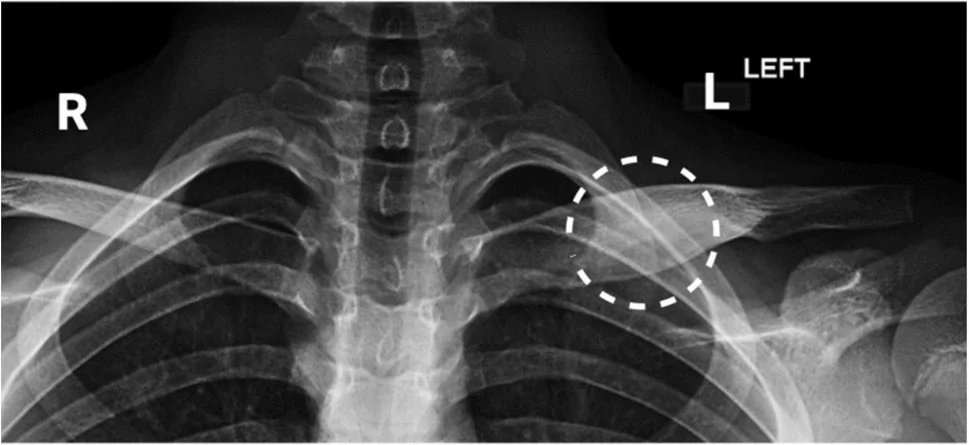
Image obtained in a 9-year-old boy with CNO involving left clavicle. Anteroposterior radiograph shows expansion of the left medial clavicle with mixed sclerotic and lytic changes (dashed circle)
